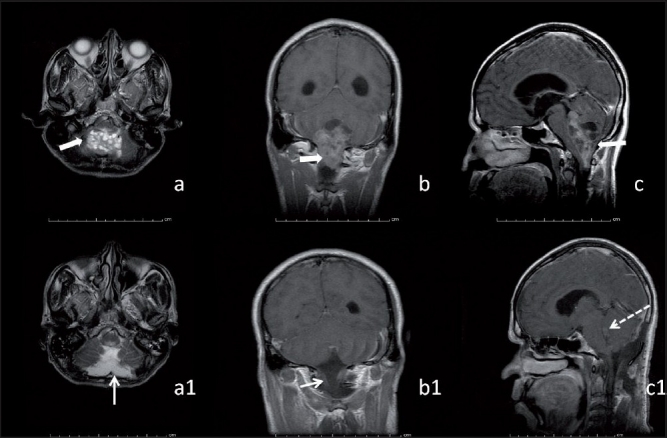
(a, b, c and a1, b1, c1): The preoperative (a, b, c) MRI of the brain compared with the postoperative MRI (a1, b1, c1). (a, a1) T2W axial images; (b, b1) axial T1W post-contrast enhancement images; (c, c1) post-contrast enhanced sag T1W images. The lesion was variegated in appearance, multicystic and revealed post-contrast enhancement. The posterior and lateral recesses of the fourth ventricle were displaced superiorly. The block arrows delineate the lesion in a–c. The thin white arrows in a1 and b1 demonstrate the postoperative cavity. The dashed arrow in c1 reveals a small residue at the floor of the fourth ventricle
